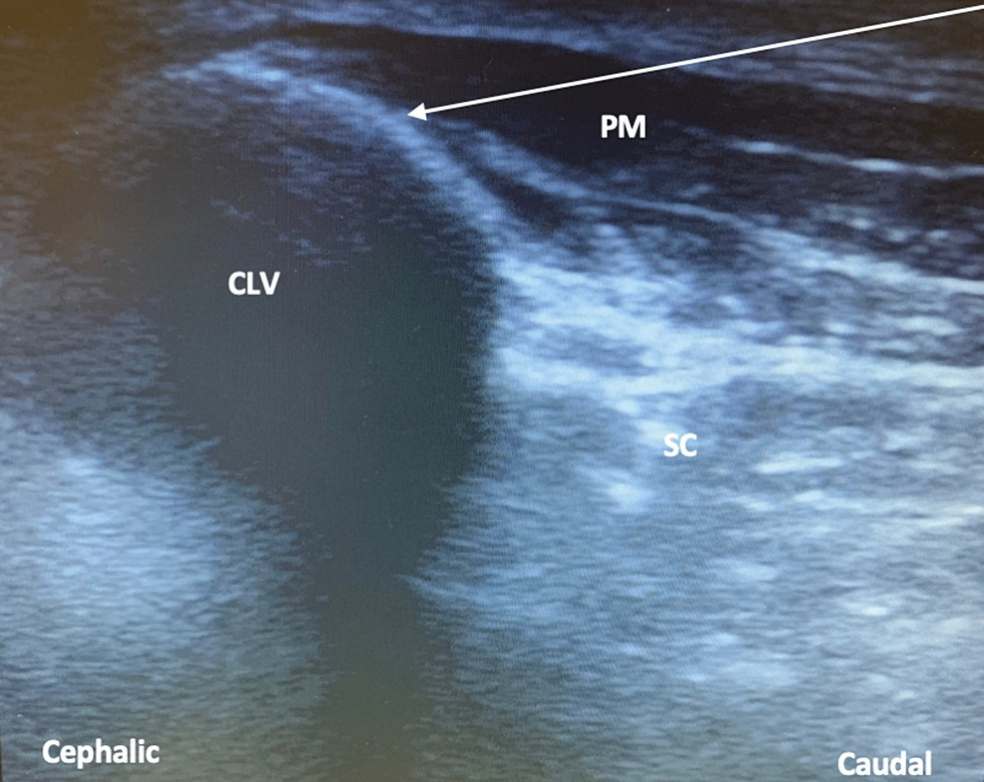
Ultrasonography image with representation of the needle and approach to the clavipectoral fascia plane block. CLV: Clavicle, PM: Pectoralis major muscle, SC: Subclavian muscle.
